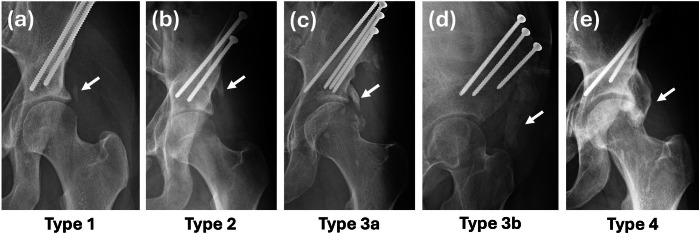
New classification system for heterotopic ossification following periacetabular osteotomy. a) Type 1 represents a small bony island, while b) Type 2 consists of a small bony spike originating from the osteotomy site. Type 3 is further divided into two subtypes: c) Type 3a is characterized by a large bony spike extending downward and laterally toward the sourcil area, while d) Type 3b involves a similar large bony spike extending downward but positioned anterior to the sourcil area, visible on a false-profile lateral radiograph. e) Type 4 denotes diffuse heterotopic bone formation around the joint line.
